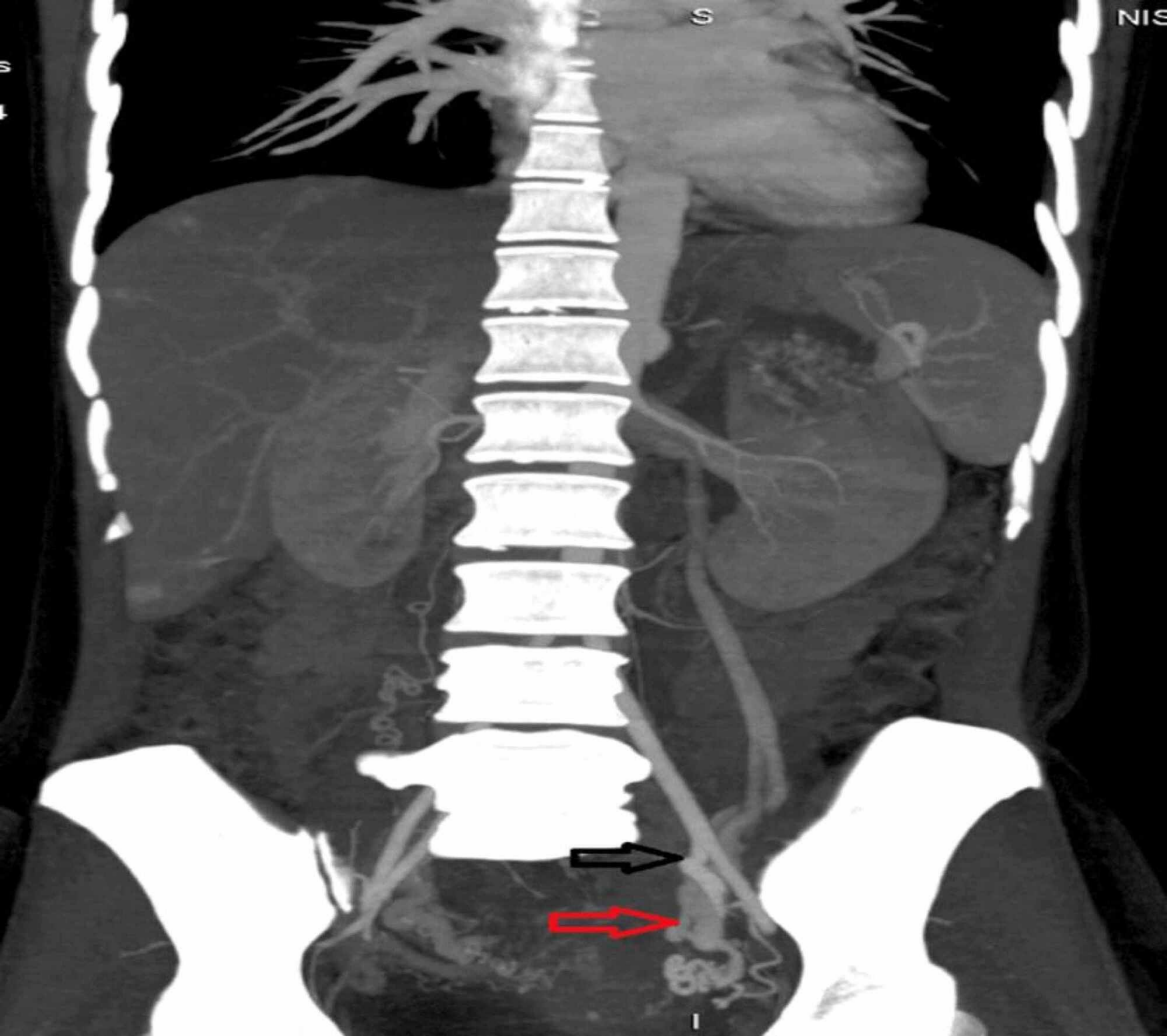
CT angiogram showing the mass to be hypervascular and fed by branches of the left internal iliac artery (black arrow) and drained by the left ovarian vein (red arrow) CT: computed tomography
